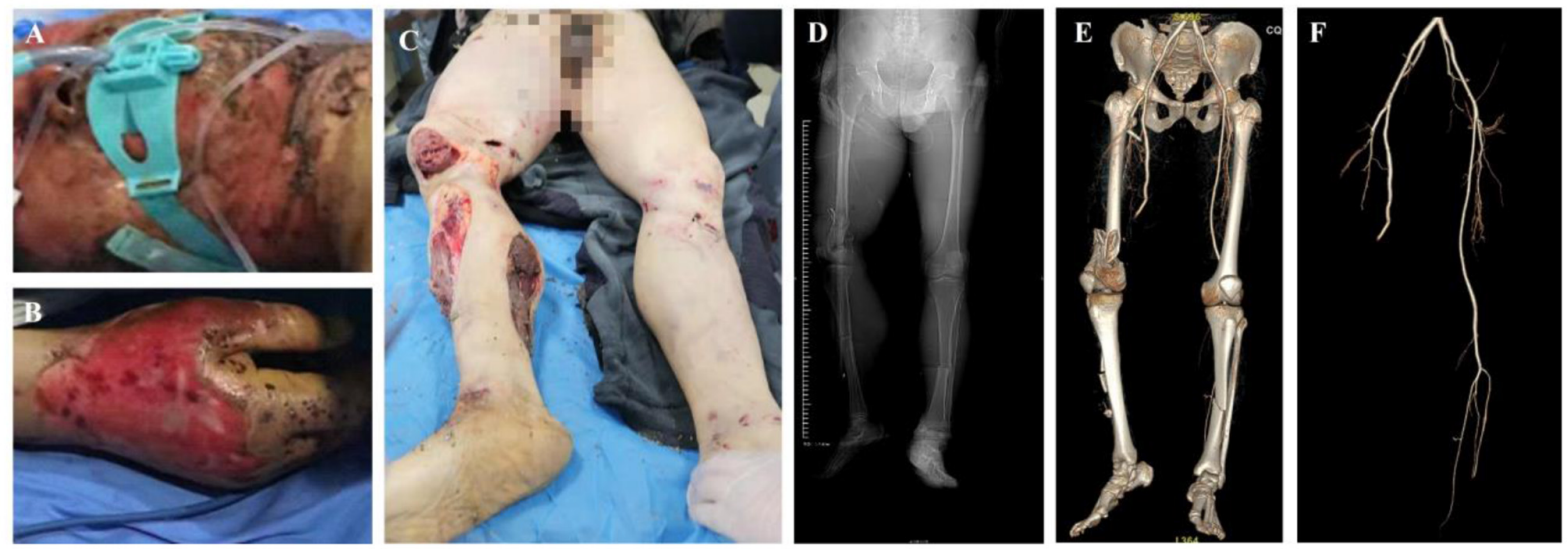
Critical Patient 9, diagnosis: blast-induced combined injury and multiple trauma (ISS 57 points: multiple-site surgeries, including amputation of the right thigh, were performed 35 h after the injury. Surgeries such as intracranial hematoma evacuation were conducted 45 h post-injury. Due to the critical condition, the patient was unable to undergo off-site imaging examinations. Subsequently, after several multidisciplinary consultations and two resuscitations, the patient died from uncontrollable intracranial hypertension and multiple organ failure on the 11th day after the injury). (A) Facial burns. (B) Hand burns. (C) Open fracture of the right lower limb. (D, E) X-ray and CT reconstruction of the pelvis and bilateral lower limbs reveals a comminuted fracture of the right distal femur and a mid-shaft fracture of the left tibia. (F) CTA of the bilateral lower limbs shows damage to the right femoral artery.
